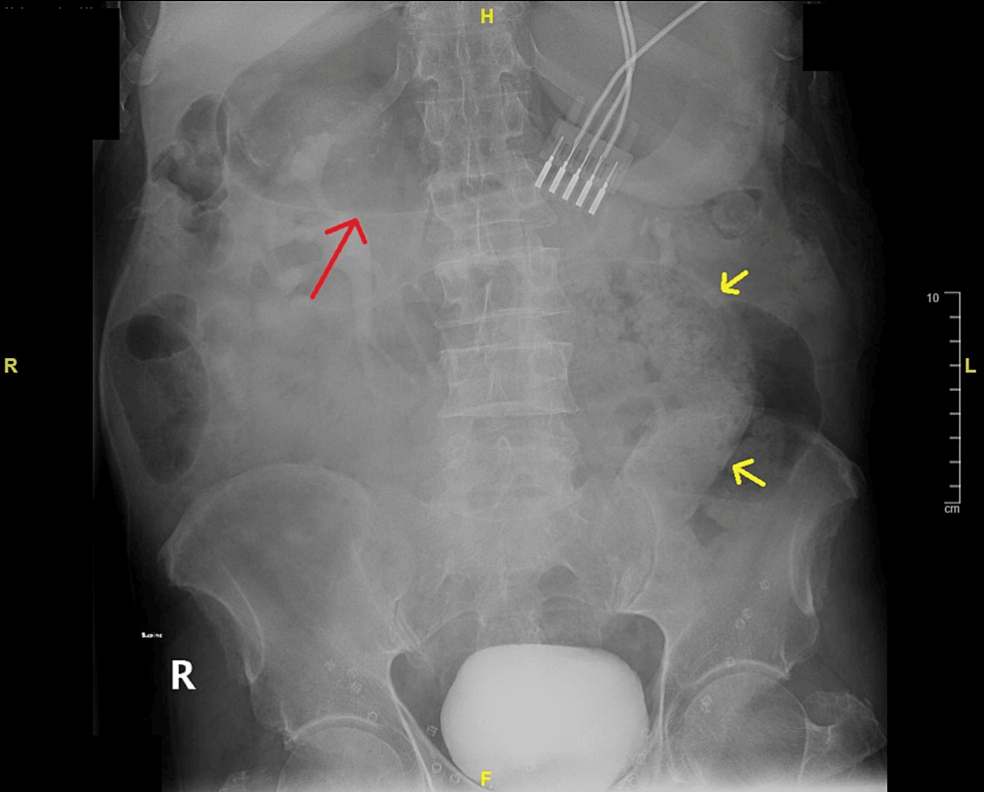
Upright abdominal X-ray showing a fecalized small bowel loop to the left of the spine (yellow arrows) with a distended stomach (red arrow)
